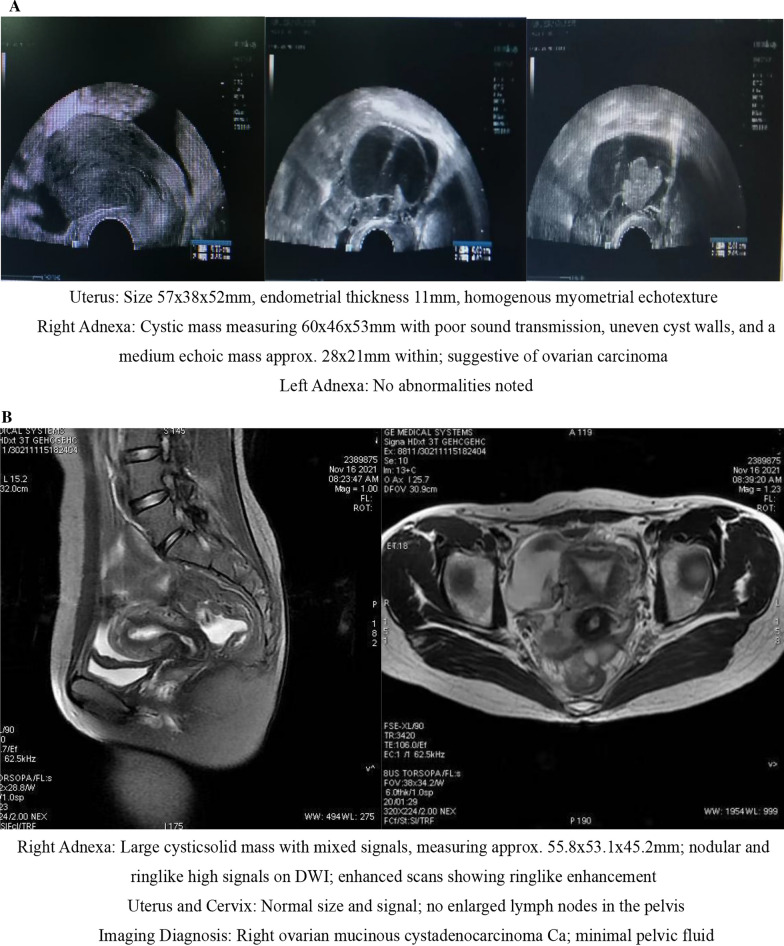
MRI Pelvis. Large cysticsolid mass with mixed signal intensity (209 × 189 × 136mm) in the lower abdomen and pelvis; multiple septations and soft tissue nodules; well-defined borders; progressive enhancement in solid components; omental thickening anterior to the mass, significantly enhancing uterus and cervix: normal signal intensity; no enlarged lymph nodes in the pelvis imaging diagnosis: ovarian mucinous cystadenocarcinoma Ca; focal omental thickening, possible metastasis not excluded
